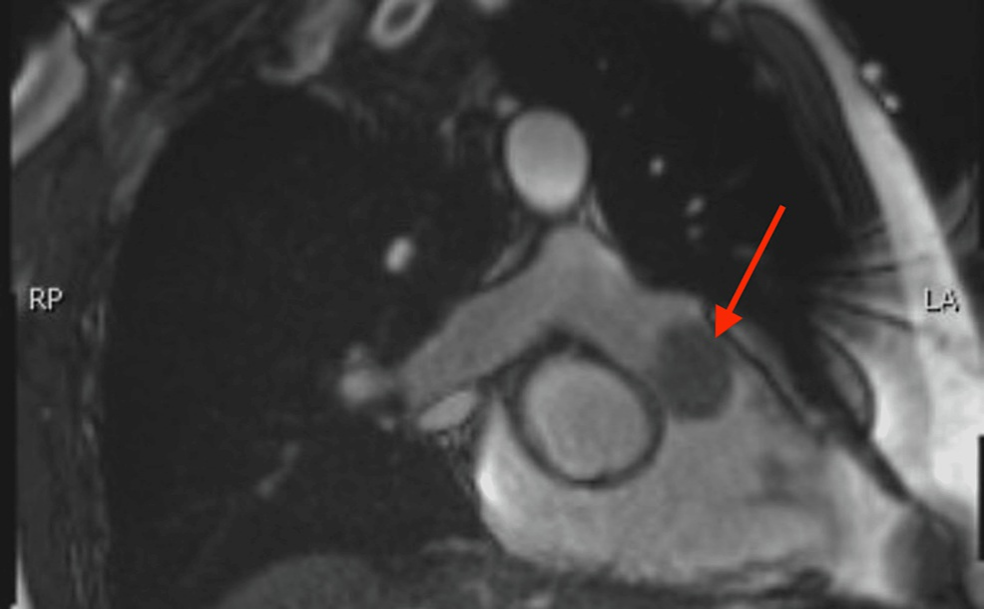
Functional cardiac MRI showing a mass attached to the pulmonic valve. MRI, magnetic resonance imaging
